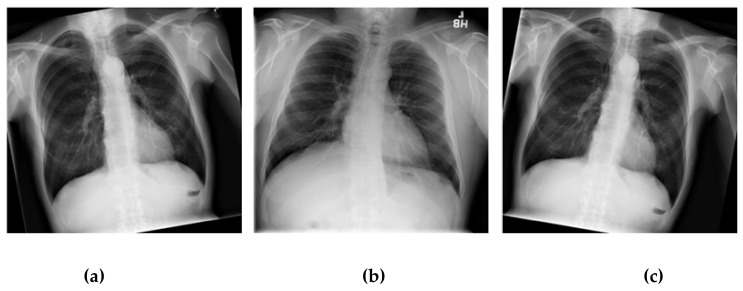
Examples of distorted chest X-ray images: (a) the patient’s normal spine tilted −10 degree by rotating; (b) a patient’s abnormal spine; (c) a patient’s normal spine tilted 10 degree by rotating.
